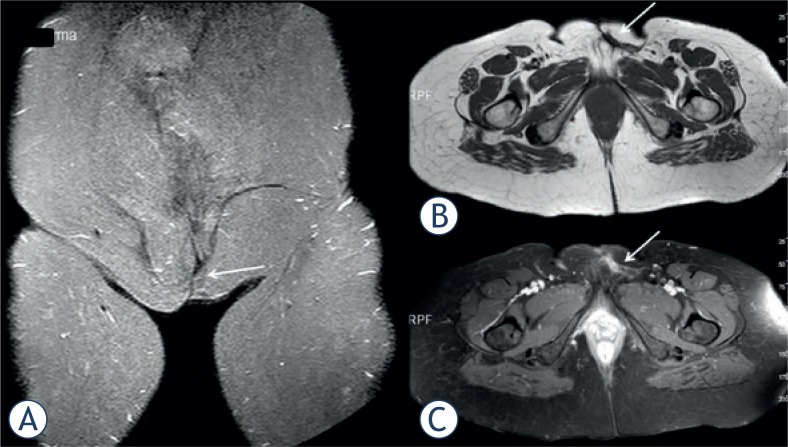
Coronal STIR A., axial T1W B., and axial contrast enhanced T1W C. MR images obtained 2 years after the surgery: postoperative changes in the operation area (arrows), no sign detected related to the recurrence.
